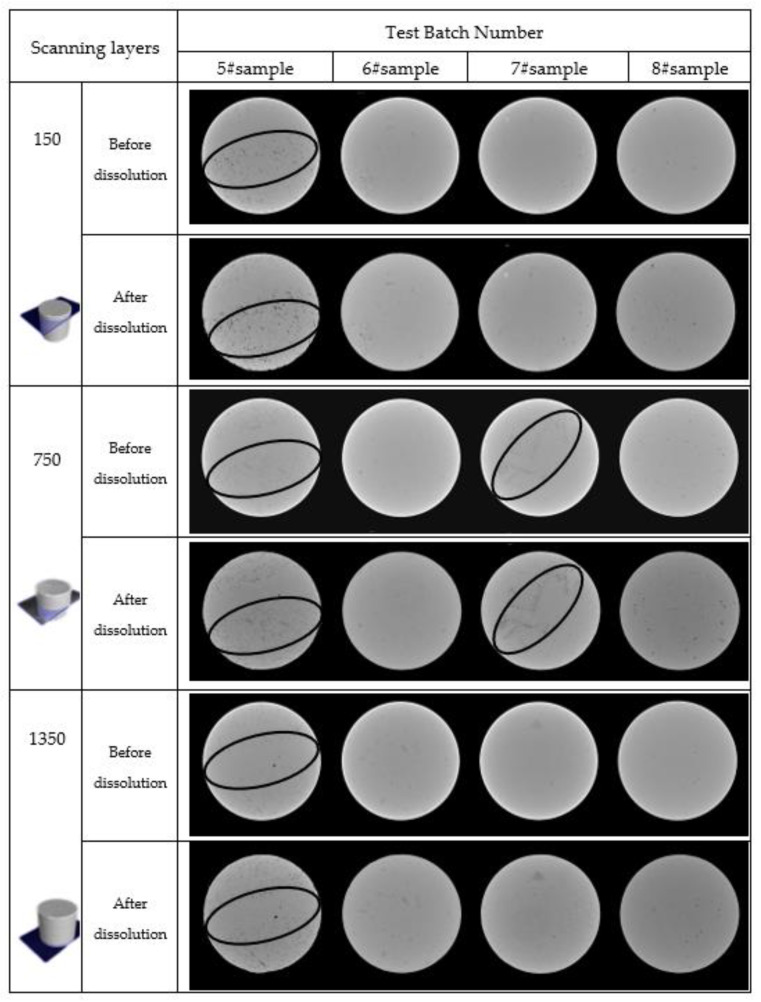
Cross-sectional view of CT scan.
